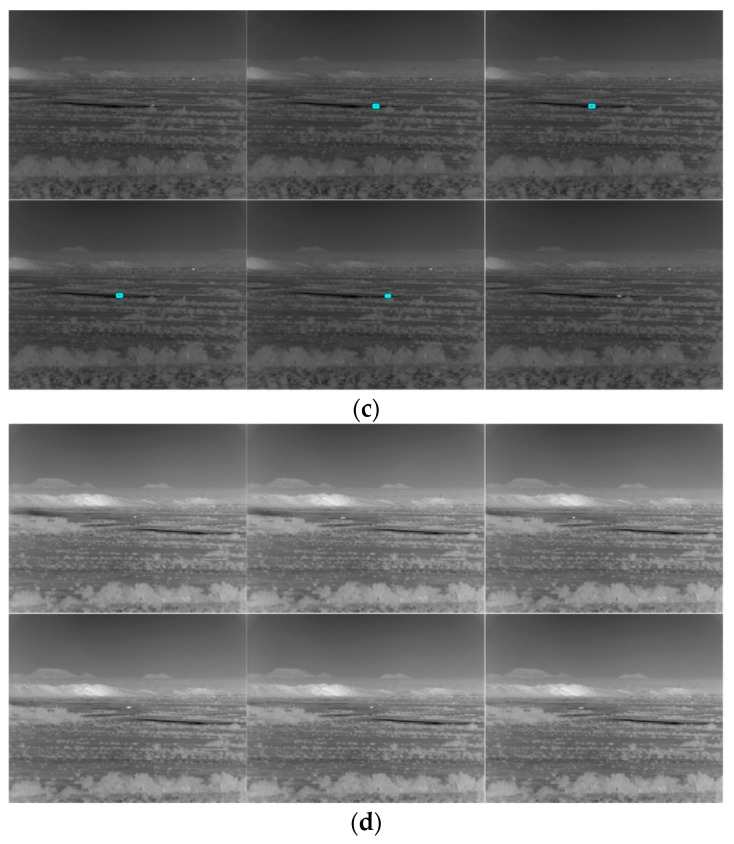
Tracking results for frames 1, 60, 119, 178, 237, and 296 for the PCE full (MWIR nighttime) case. The vehicle is SUV. (a) 1000 m; (b) 2000 m; (c) 2500 m; and (d) 3500 m.
